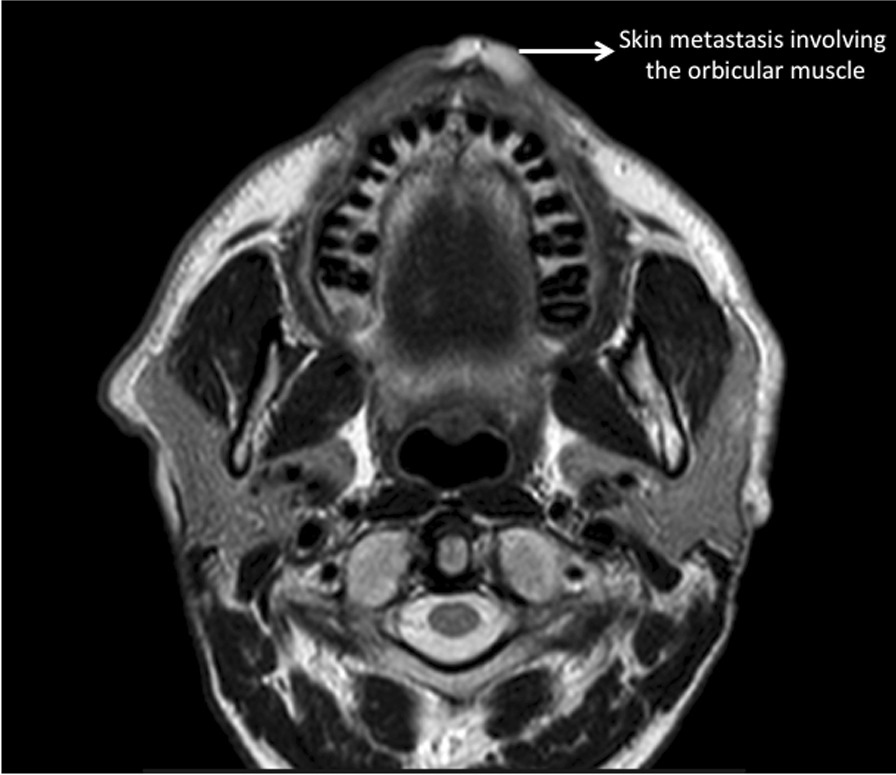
Facial MRI showing an involvement of the orbicular muscle by the metastatic process
